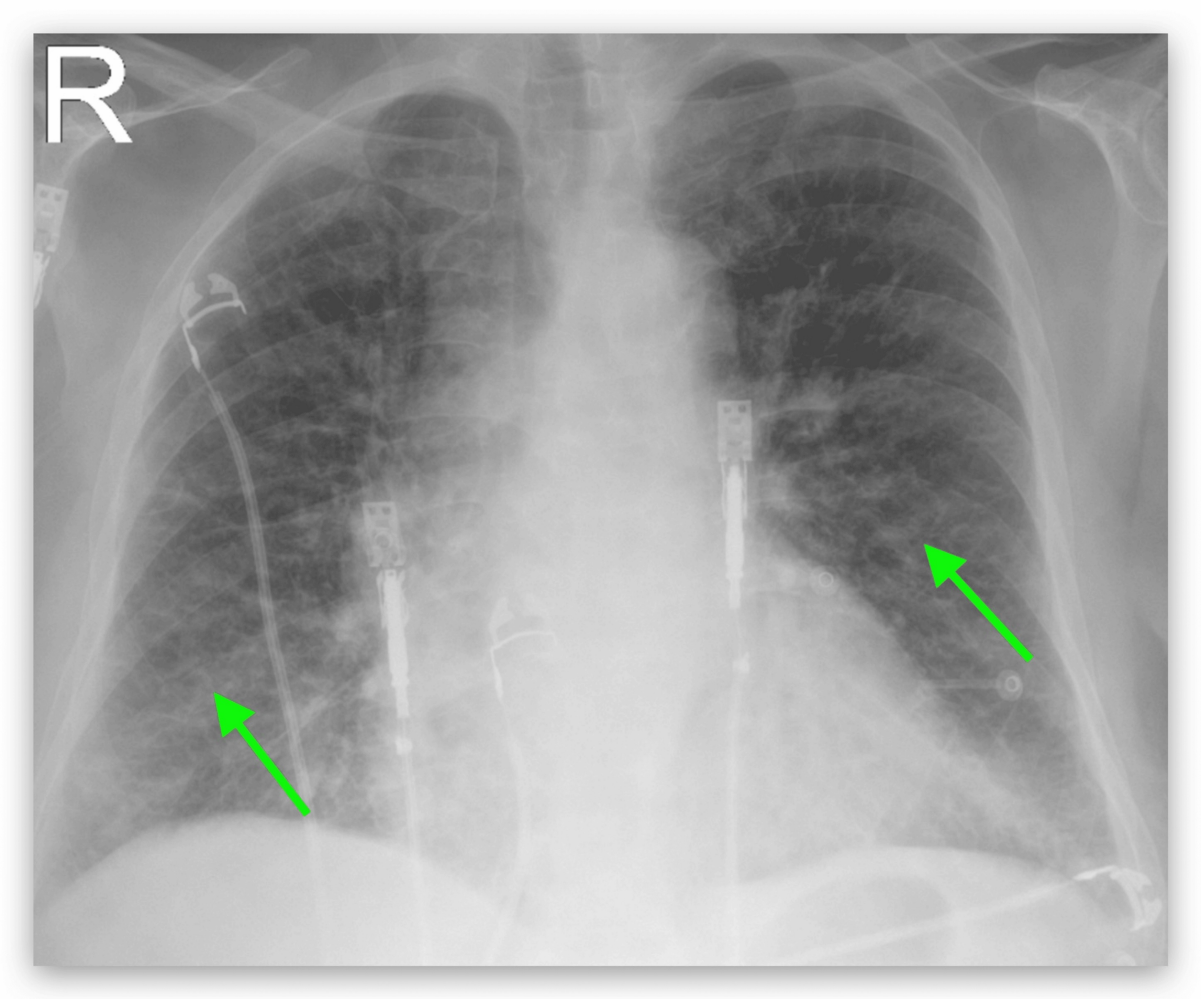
Chest X-ray showed diffuse interstitial infiltrates (green arrows)
